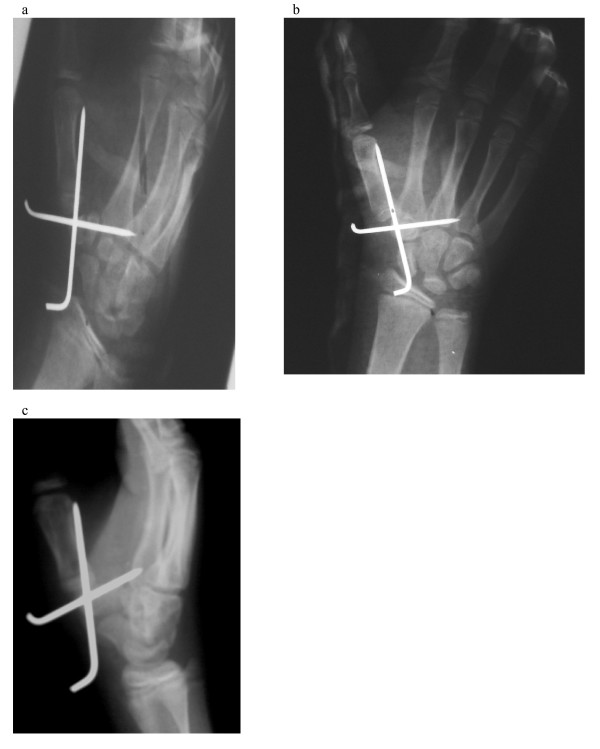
Radiographs of the same patient after open reduction of the fracture dislocation and stabilization with K wires.
